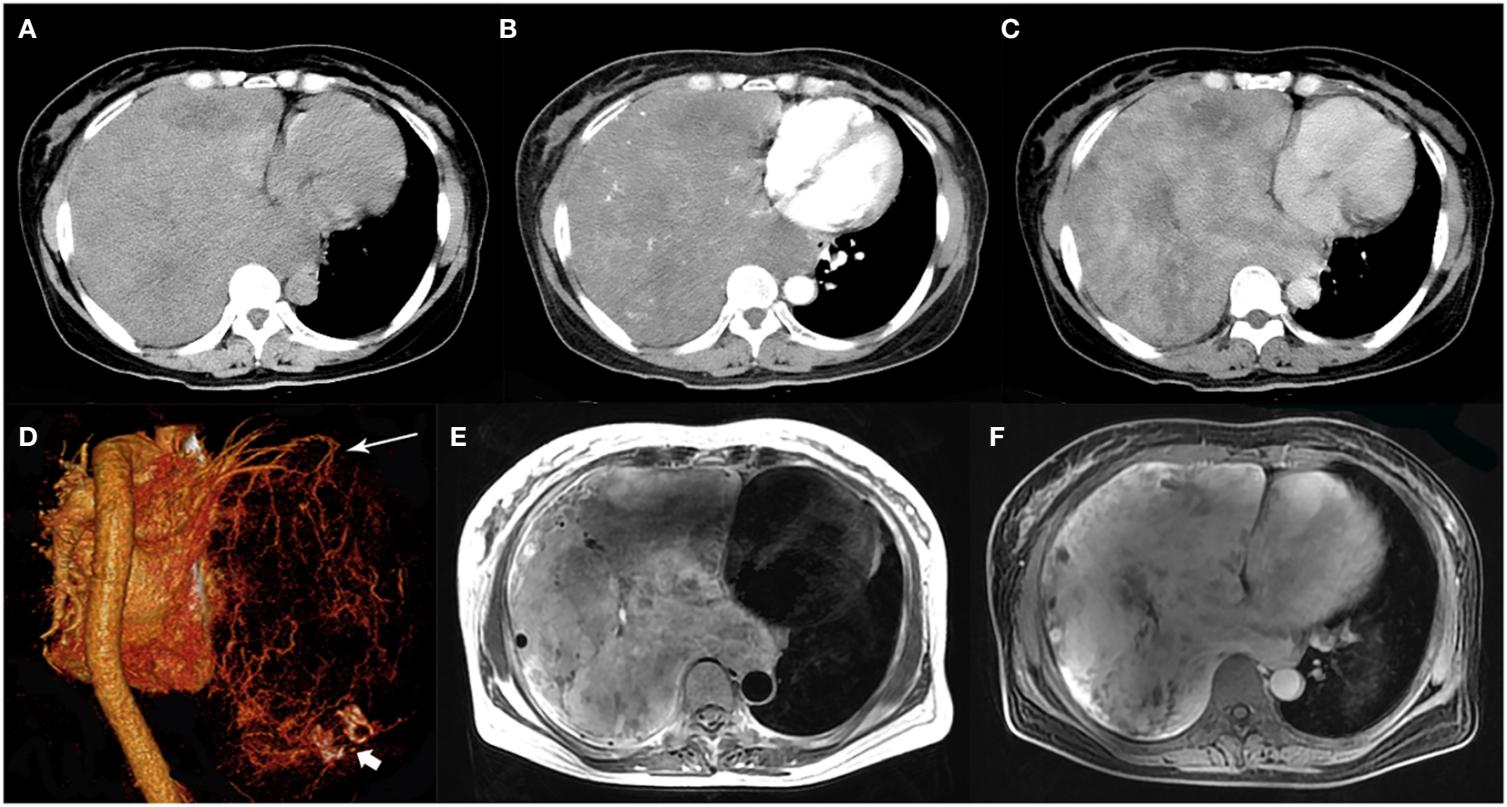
A 51-year-old woman with SFTP. (A) Precontrast CT scan shows a huge mass with relatively high density in the right thoracic cavity. (B) Numerous enlarged intratumoral vessels are seen in the arterial phase on post-contrast CT image. (C) Heterogeneous marked enhancement is detected in the venous phase. (D) Collateral feeding arteries arising from the right upper pulmonary artery are detected on 3D VR image (white arrow). Intratumoral calcification is also detected (white arrow head). (E) A black-and-white mixed pattern and multiple flow voids are seen on T2-weighted image. (F) Heterogeneous moderate to marked enhancement are seen on postcontrast MR image.
